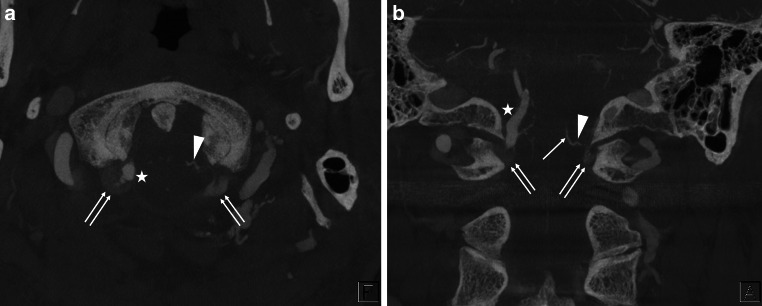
A representative case of the suboccipital cavernous sinus (SCS) bridging vein (BV). Axial (a) and coronal (b) view of the slab MIP images from contrast-enhanced cone-beam CT show that the lateral medullary vein (*arrow* in (b)) drains into the SCS (*double*
